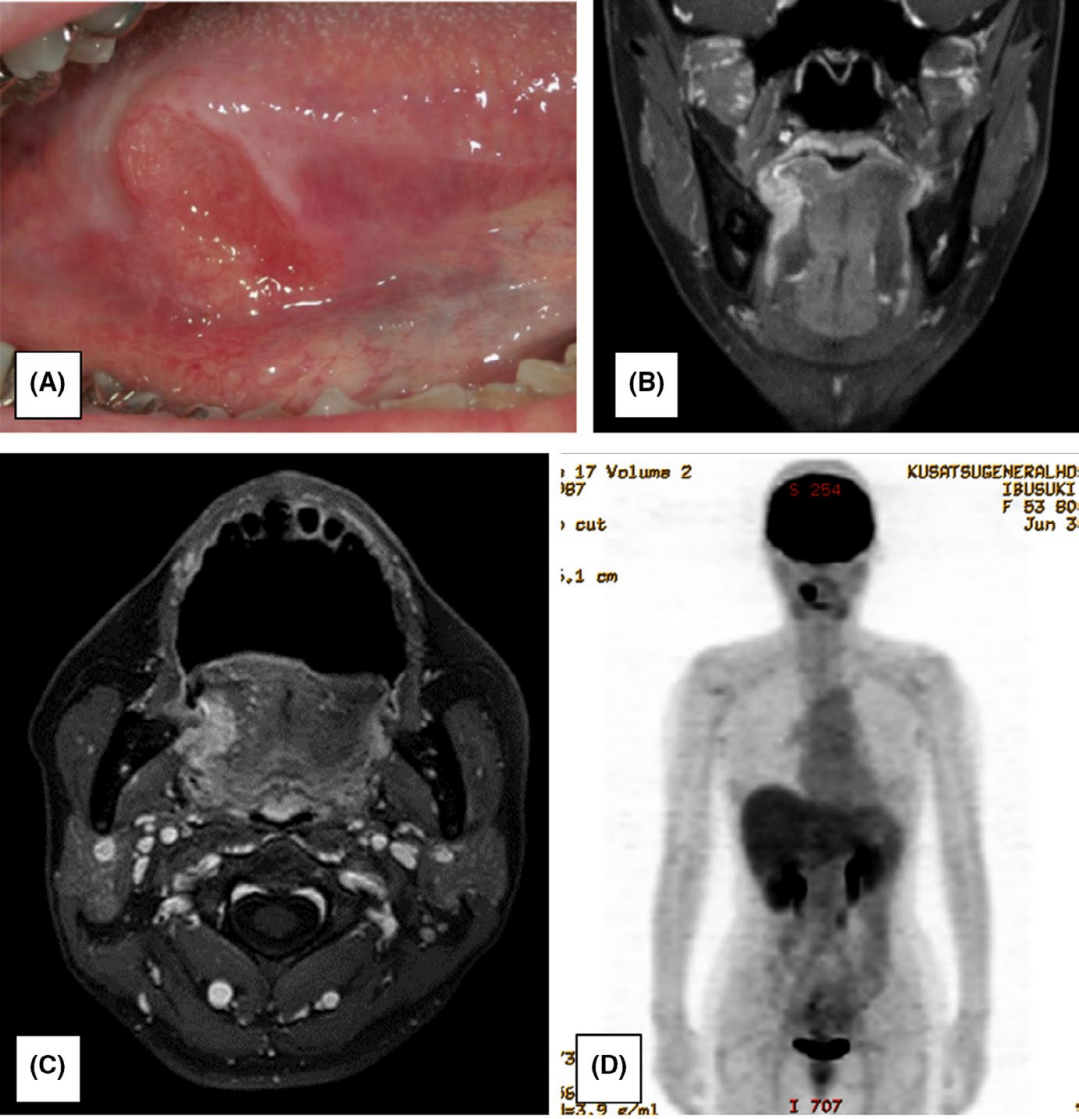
(A) Photograph taken during the patient's initial visit, showing an ulcerative lesion measuring approximately 22 mm in diameter on the right tongue margin. (B) Magnetic resonance image showing frontal disconnection. (C) Magnetic resonance image in the horizontal view. An invasive lesion showing a contrast effect with gadolinium was observed on the right tongue margin. (D) Positron emission tomography image showing a high accumulation of fluorodeoxyglucose in the right tongue margin
